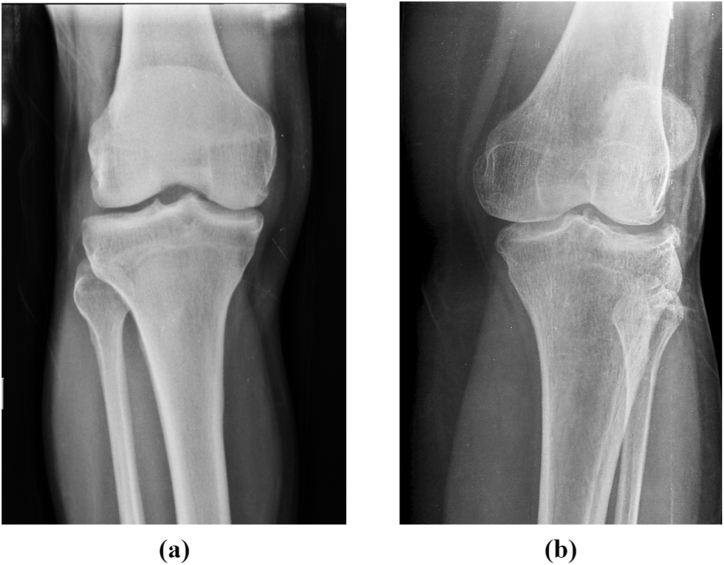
Open-source Knee-Xray scans: (a) Depict the normal patient, (b) Depicts the scan of an Osteoporosis patient (Steven [2]).
